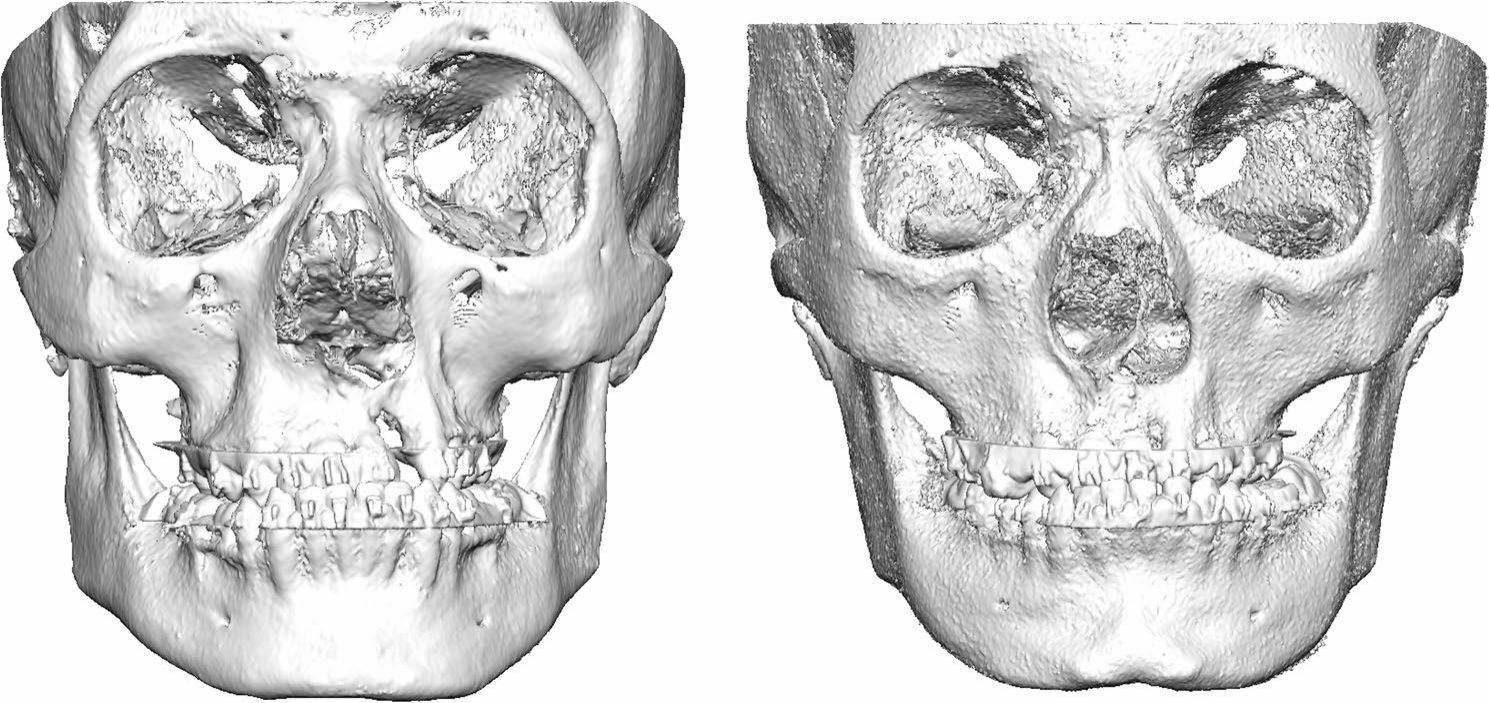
Preoperative frontal view before Le Fort I osteotomy. Left: CBCT image acquired using the standard-dose FACE protocol. Right: CBCT image acquired using the ULD JAW protocol
